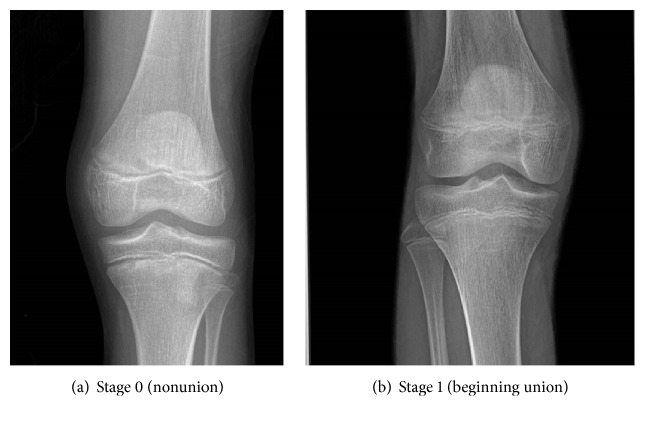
Radiographic images of knee joint showing stage 0 labeled as (a) and stage 1 labeled as (b).
